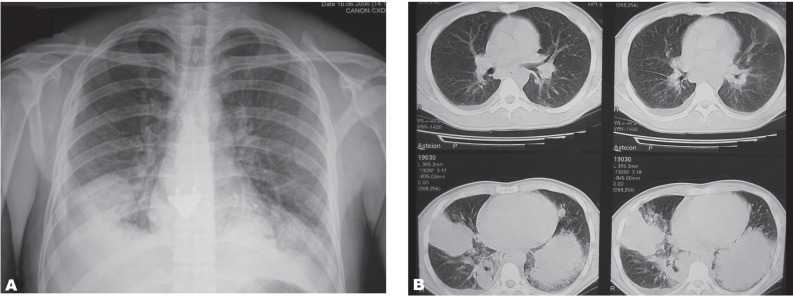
A 29-year-old male patient with fire eater’s pneumonia. (A. Partial opacities eliminate the heart and diaphragmatic contours in the bilateral lower zones in his chest radiography. B. Minimal pleural liquid is seen on the right side with dense and solid parenchymal masses in the bilateral lower lobes, density increases in the form of ground glass together with pulmonary nodules in thoracic CT.)
